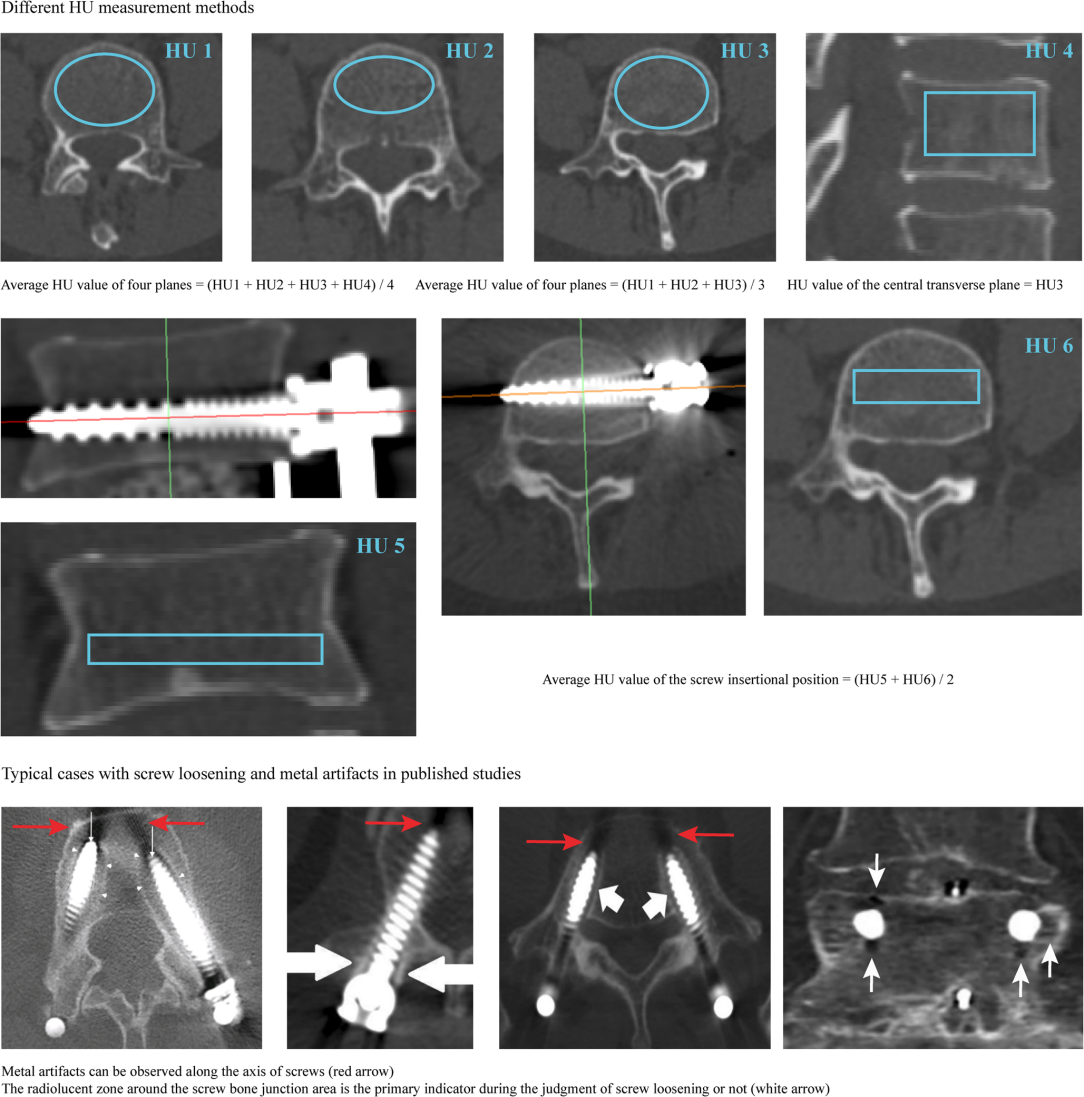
Different HU measurement methods and the judgment of screw loosening status by CT imaging data (Metal artifacts can be observed along the axis of the screw, and the radiolucent zone around the screw bone junction area is the primary indicator during the judgment of screw loosening or not) [5, 27–29]
